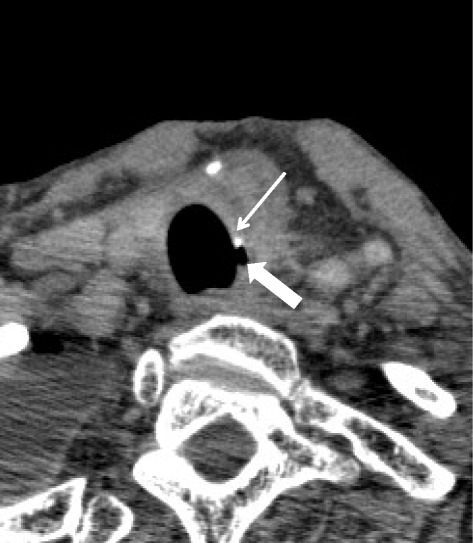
CT scan showed that a fistula (thick arrow) had developed on the left side cartilage portion of the trachea. A circular stapler (*thin arrow*) was also found at the fistula portion
